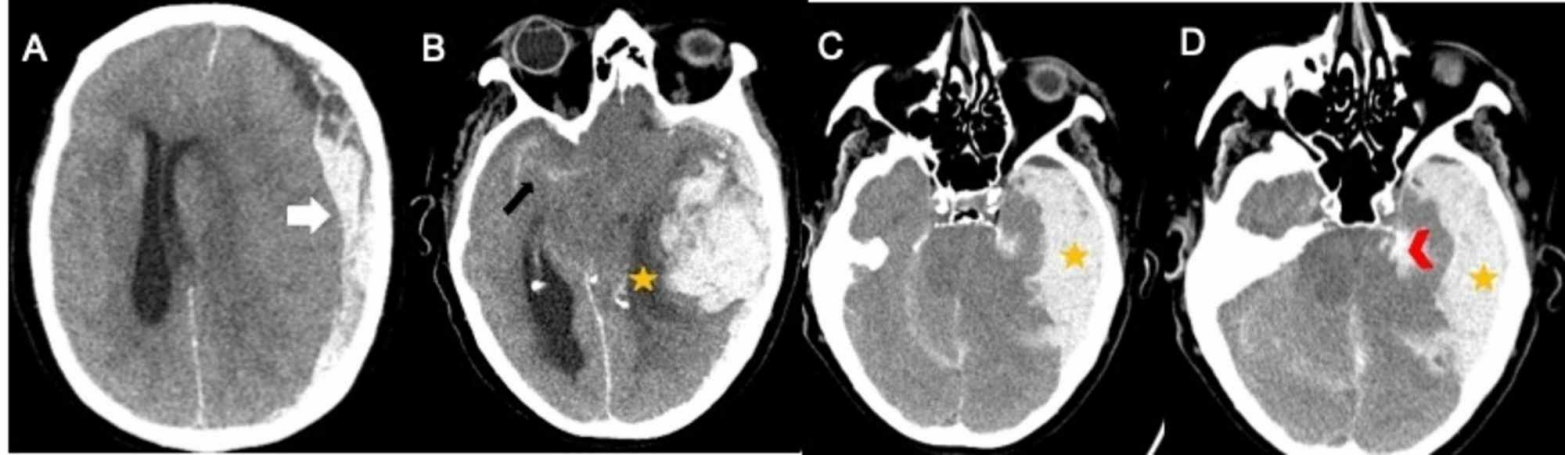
CT of the head without contrast (image A) shows crescent-shaped hyperdensity (white arrow in image A) on the left frontoparietal cortex with midline shift suggesting hyperacute and active hemorrhage. Images B-D show hyperdensity in the right Sylvian fissure consistent with subarachnoid hemorrhage (black arrow in image B) and intraparenchymal hematoma in the left temporal lobe with surrounding hypodensity (yellow star in images C and D) causing severe transtentorial herniation (red arrow in image D).
